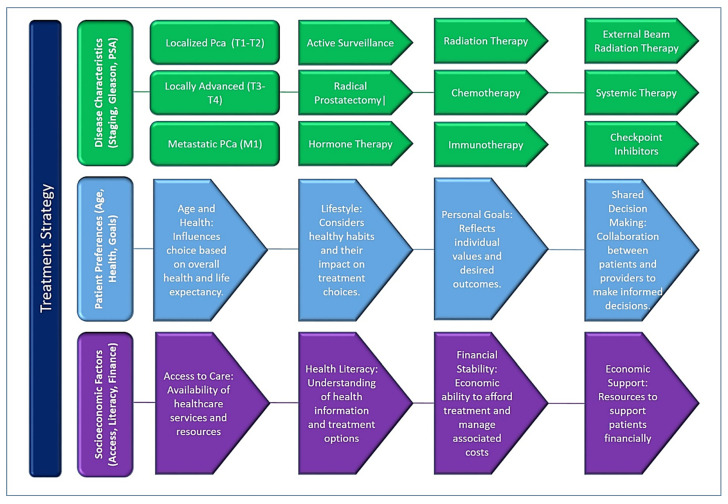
A graphical scheme representing the key factors influencing treatment decisions in prostate cancer, emphasizing the interplay between disease characteristics, patient factors, and healthcare system considerations. Treatment strategies are tailored based on the stage of cancer (localized, locally advanced, or metastatic), Gleason score, PSA levels, PSA density (PSAD), and imaging findings such as multi-parametric MRI (mpMRI). These clinical parameters guide whether patients are managed with active surveillance, surgery, radiation, systemic therapies, or palliative care.
